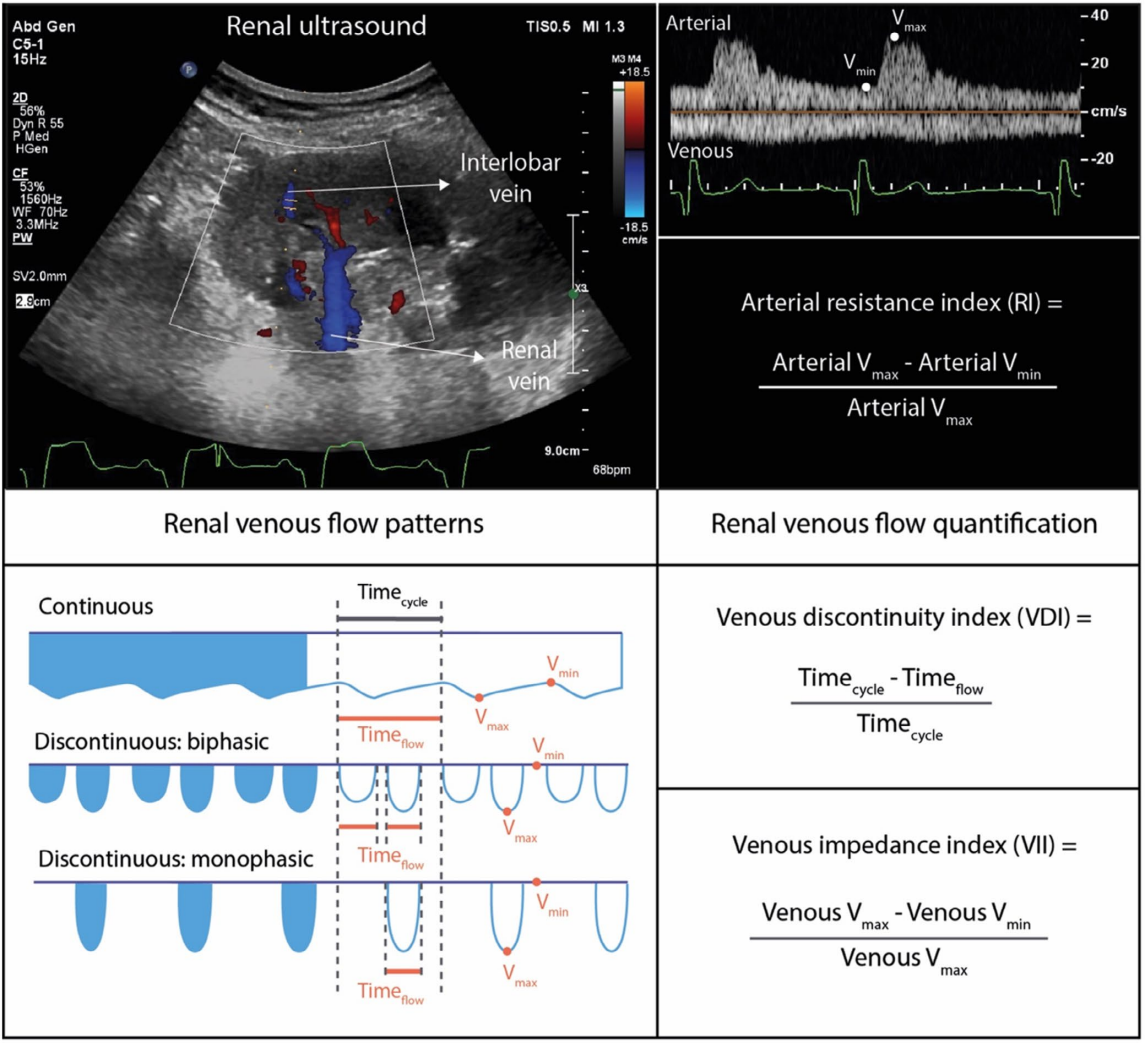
Assessment and calculation of renal ultrasound parameters. RRI = intrarenal resistance index, VDI = venous discontinuity index, VII = venous impedance index. Reprinted from: The Effect of Decongestion on Intrarenal Venous Flow Patterns in Patients With Acute Heart Failure, Journal of Cardiac Failure, Volume 27, Issue 1, January 2021 (with permission).
